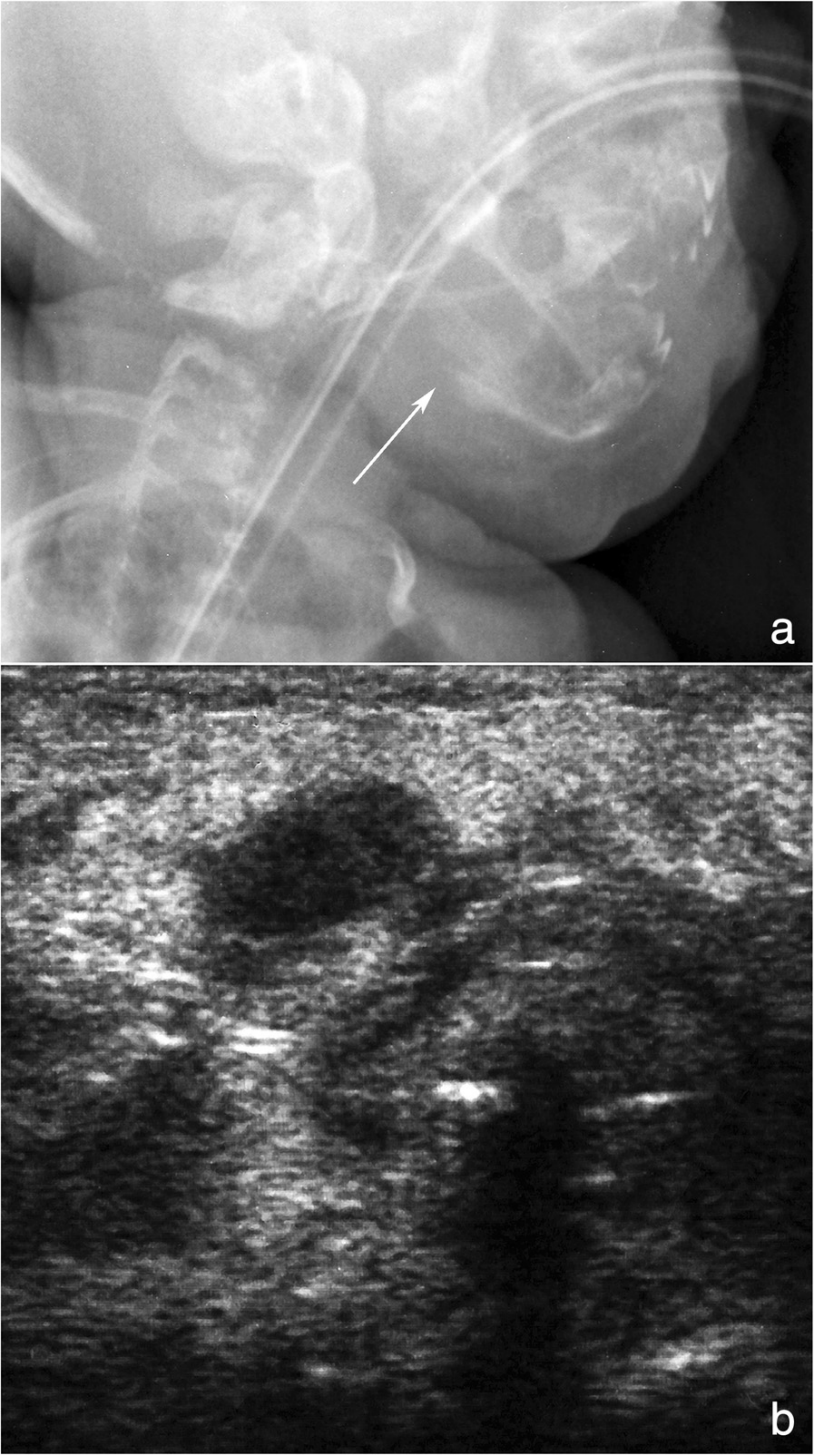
X-ray and ultrasound evaluations. Facial bone X-rays in lateral projection (a) showed the osteolytic disruption of the right horizontal mandibular ramus with focal periosteal reaction and swelling of the surrounding soft tissues. The ultrasound examination (b) confirmed X-ray findings and also demonstrated a hypo-anechoic central image consistent with initial abscess collection
